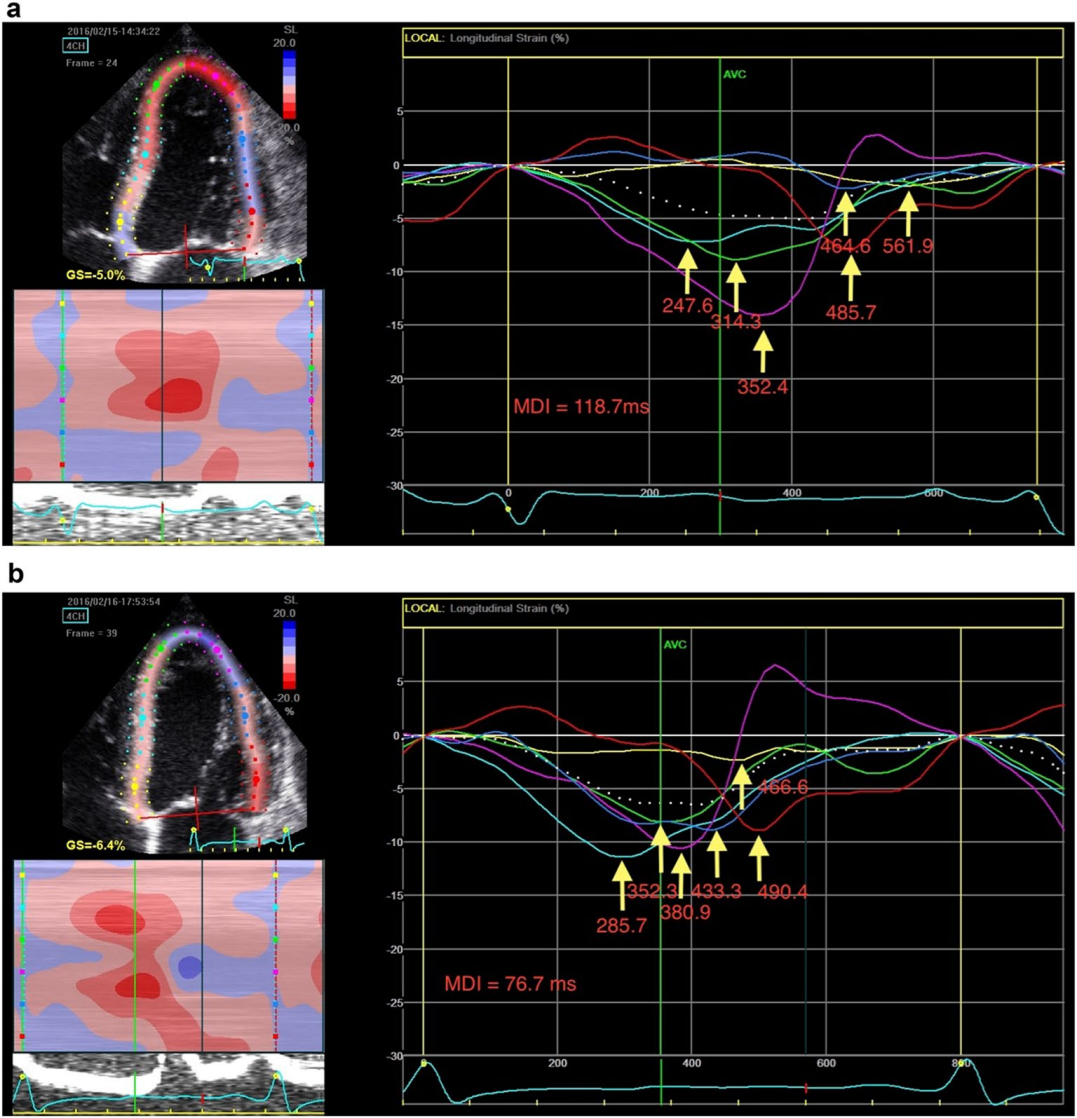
Strain tracings from an apical 4 chamber view of a patient with acute heart failure at: a baseline and b 23-h follow-up. Each yellow arrowhead points to the peak longitudinal strain for one of the 6 segments of the left ventricular wall visualized in this view. The values adjacent to each arrowhead represent the time-to-peak longitudinal strain for the corresponding segment. Mechanical dispersion index (MDI) is calculated by calculating the standard deviation for the time-to-peak longitudinal strain values. After 23 h of treatment, the MDI has improved (decreased) by approximately 35% in this patient who had a favorable post-discharge outcome
